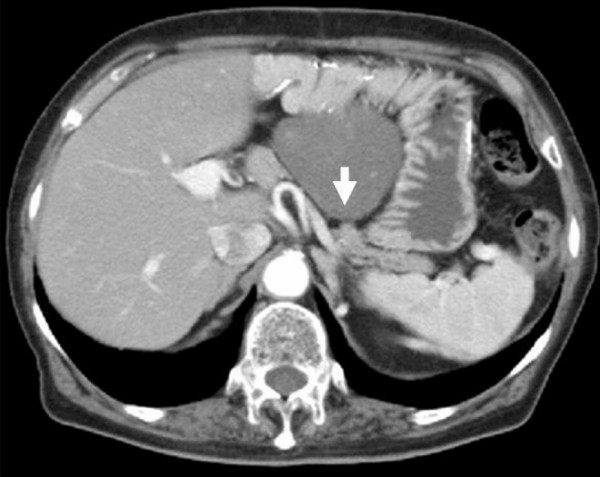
Computed tomography (CT) shows round-shaped solid mass at the right side of the reconstructed jejunal pouch, and invaded into pancreas (arrow).
